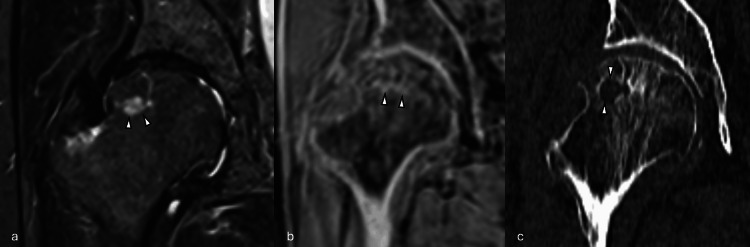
Representative imaging example 5. A false-positive case by pseudo-CT. (a) short tau inversion recovery MR, (b) pseudo-CT, and (c) CT. An intraosseous cyst (arrowheads) at the right femoral head was incorrectly interpreted on pseudo-CT as a fracture by one of the readers.
